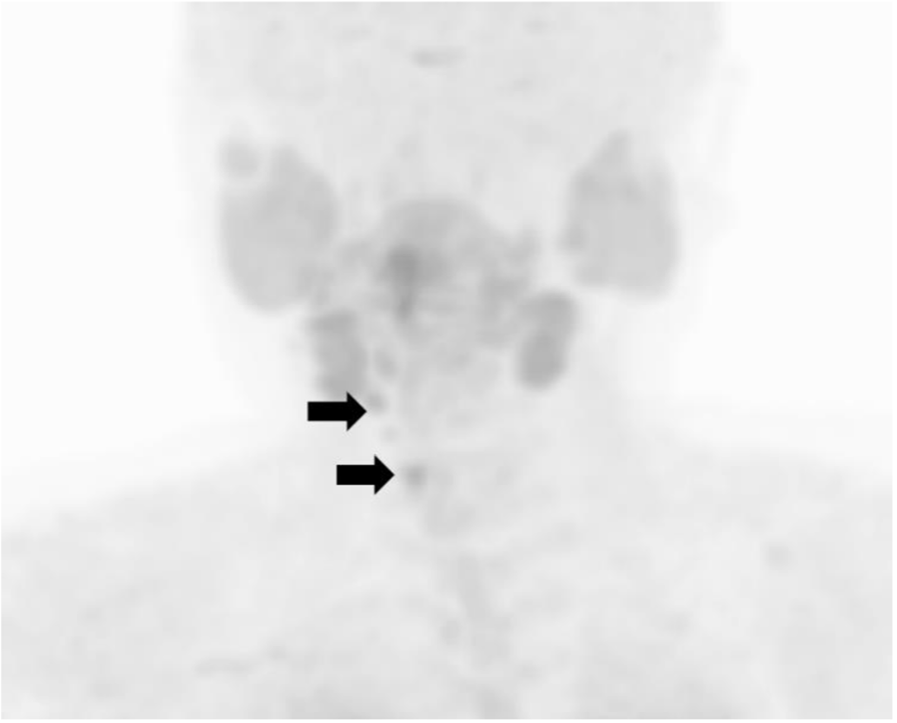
^11^C-methionine PET-CT findings after the removal of local recurrence. Methionine-positive tumors right next to the trachea (arrows)
